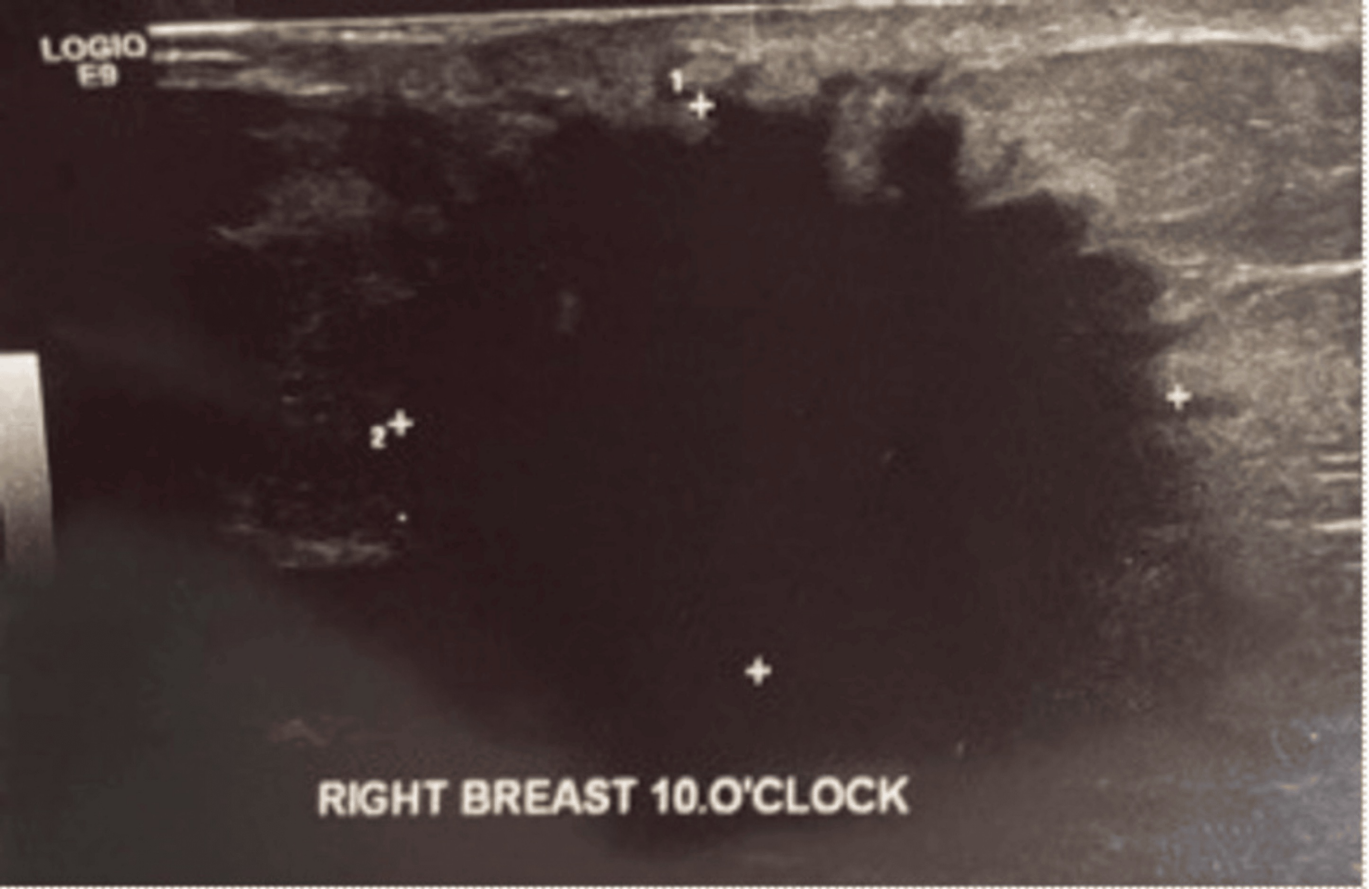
Ultrasonography of the 2.8 cm right breast heterogenous mass with irregular borders
